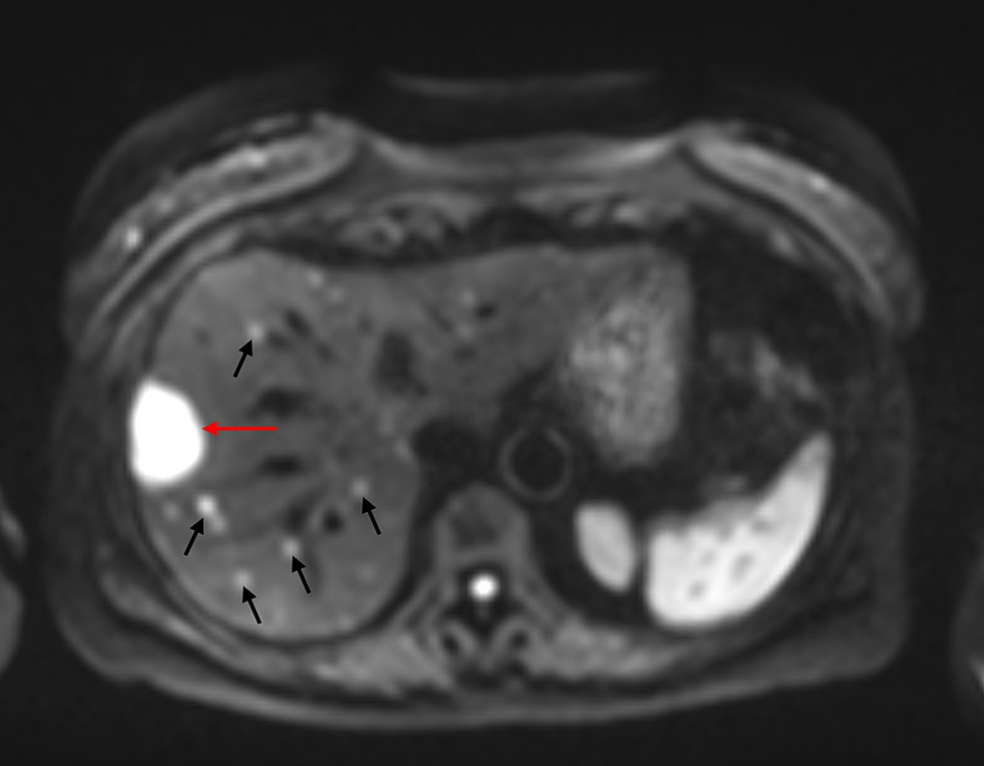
MRI liver with hepatobiliary contrast Diffusion-weighted imaging of the liver showing dominant metastasis in segment 8 measuring 3.6 cm (red arrow) and multisegmental subcentimeter metastases (black arrows).
